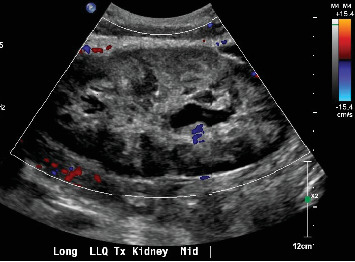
Transverse ultrasonographic image of a renal allograft. Despite the application of appropriate low-flow Doppler settings, no detectable blood flow was observed within the renal graft parenchyma or the principal renal vasculature, suggestive of graft thrombosis or severe ischemia.
